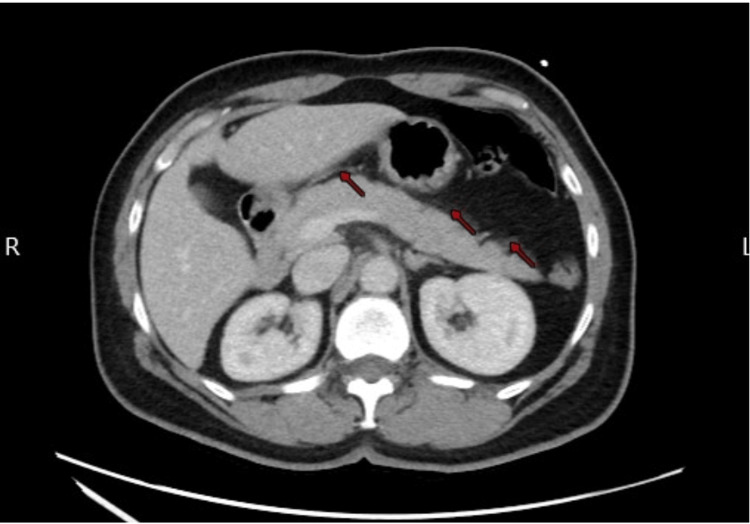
CT evidenced the pancreatic fatty stranding.
